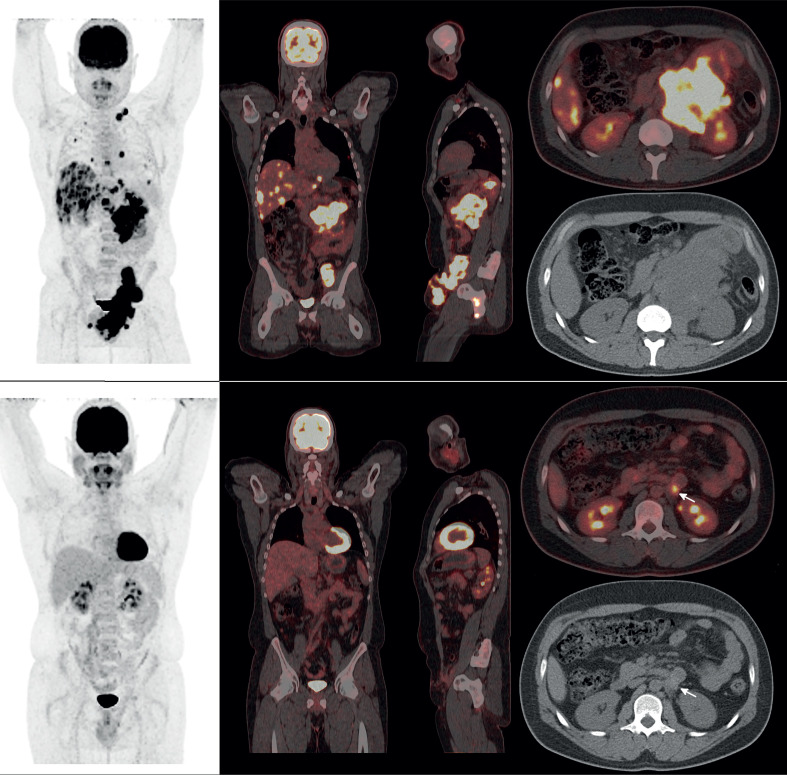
Upper panel: Postoperative 18F-FDG PET/CT scan (MIP and fused coronal, sagittal and axial slices) revealed extensive scrotal-inguinal, pelvic, mesenteric, retroperitoneal, hepatic, pulmonary, supradiaphragmatic and skeletal dissemination, with high metabolic-rate (SUVmax: 22.7). Lower panel: Post-treatment 18F-FDG PET/CT (MIP and fused coronal, sagittal and axial slices) revealed an almost complete metabolic remission after four cycles, with the persistence of retroperitoneal minimal residual disease (arrows, SUVmax 6.1) that are candidates for surgical or radiotherapy treatment.
